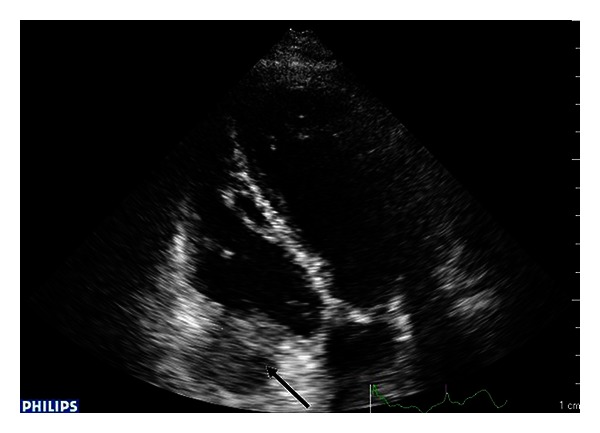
Hypoechoic mass involving tricuspid annulus. Echocardiography apical four chambers view showing a hypoechoic mass involving tricuspid annulus and right ventricle outflow tract (arrow).
